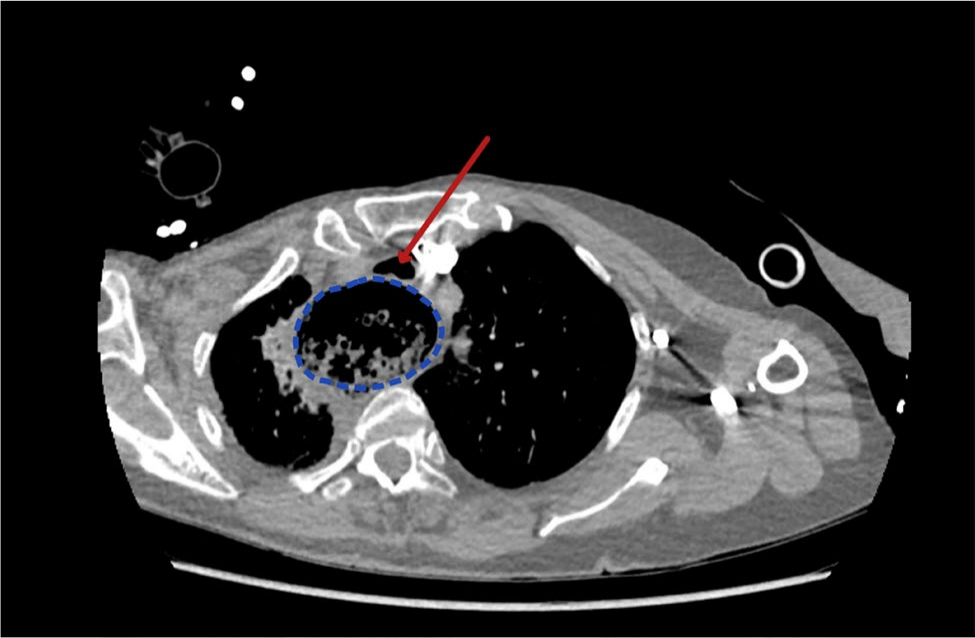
Axial view of CT pulmonary angiogram taken on admission showing a grossly dilated oesophagus (circled in blue) with compression of the trachea (highlighted by red arrow)
